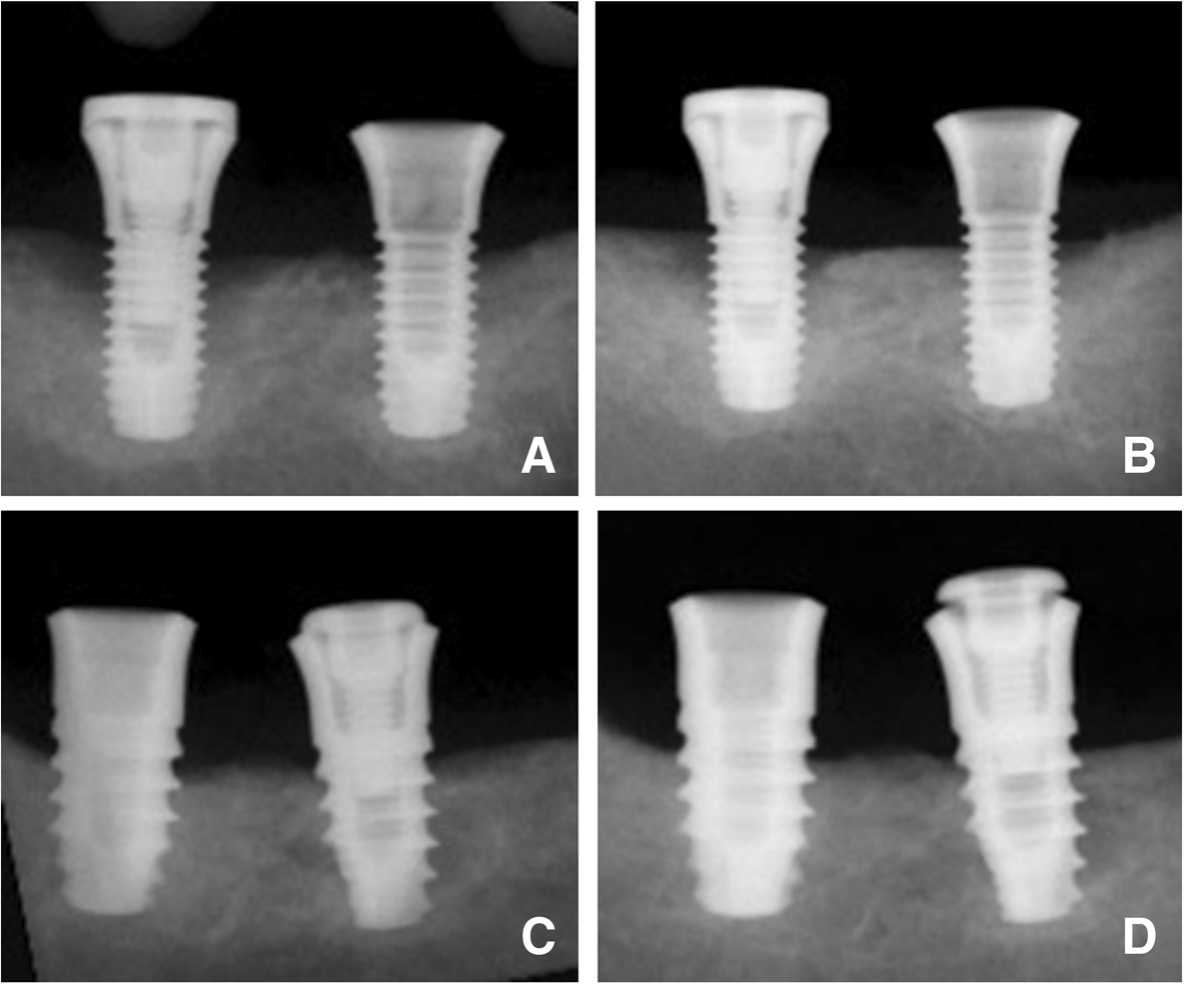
X-ray images. a, b Implants 3.3 mm in diameter (a) at the time of treatment and (b) after 5 months of healing. c, d Implants 4.25 mm (at the left) and 3.8 mm (at the right) in diameter (c) at the time of treatment and (d) after 5 months of healing
